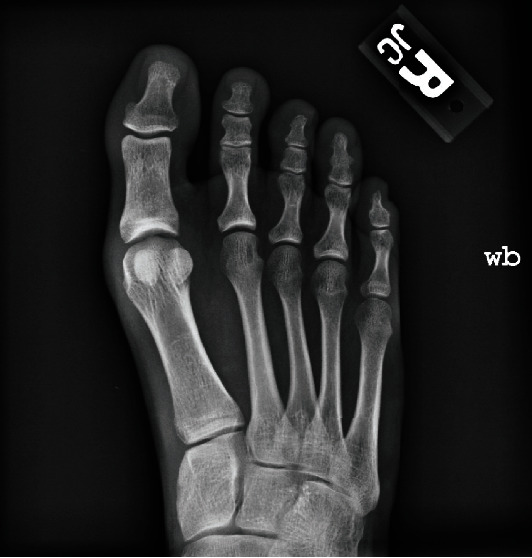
Preoperative right foot anteroposterior radiograph taken at the time of presentation.
